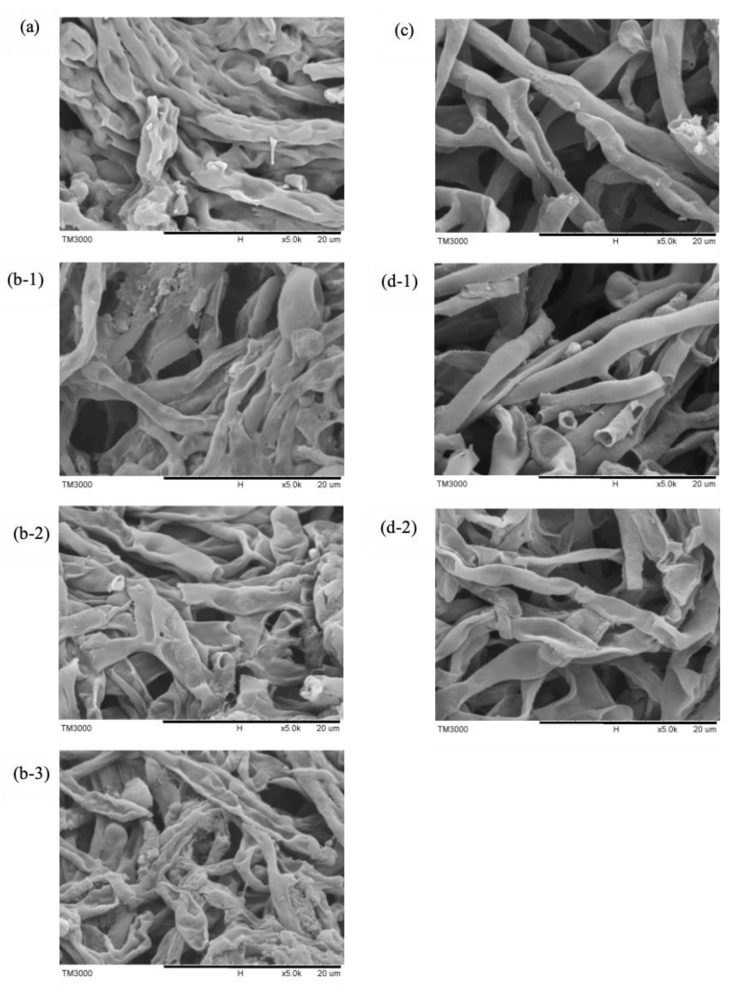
SEM images of *M. alpina* FU30797 in 5k magnitudes, where: (a) untreated biomass, (b) HCl treatment: 1 M (b-1), 2 M (b-2), 3 M(b-3), (c) ultrasonication 20 W and (d) HPP (600 MPa, 1 time) by water medium(d-1) and ethanol medium(d-2).
